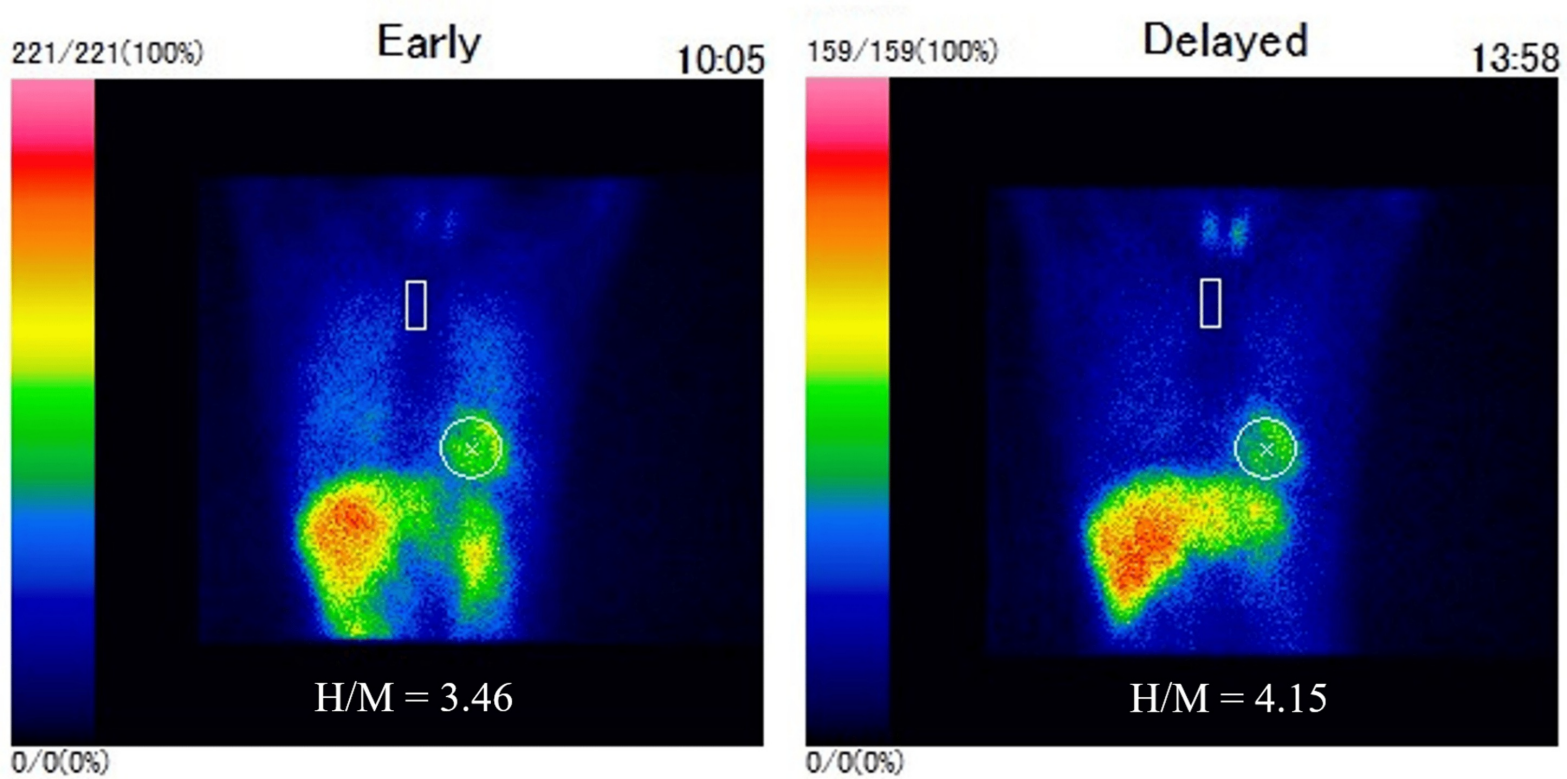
123I-metaiodobenzylguanidine (MIBG) myocardial scintigraphy findings. MIBG myocardial scintigraphy showed normal accumulation of the nuclide in the heart with a heart/mediastinum (H/M) ratio of 3.46/4.15 (early/delayed).
